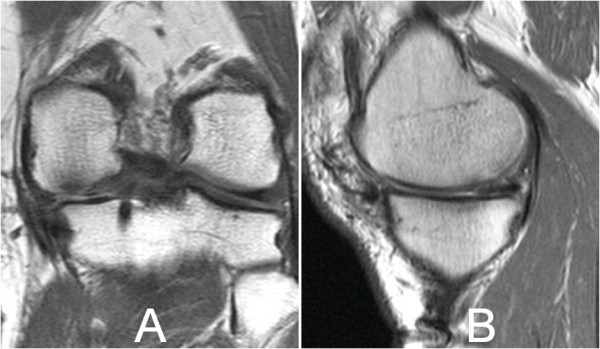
MRI in T2 at final follow-up. MRI in coronal (7A) and parasagittal (7B) planes in T2 sequences showing the correct fitting of the meniscal complex implantation at 24 months. In the coronal plan (7A) size and shape are preserved at last follow up. A mild anterior extrusion is seen in the sagittal plane (7B).
